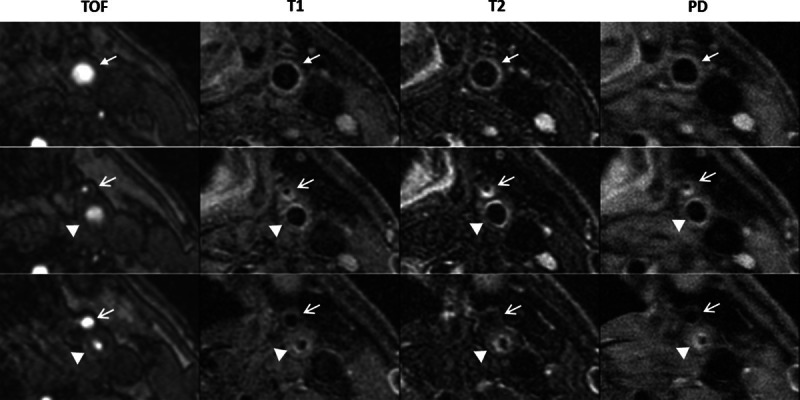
Multisequence MRI of the internal carotid artery at 3 different levels: first column; time-of-flight (TOF), second column; T1-weighted turbo-spin echo (T1), third column; T2-weighted turbo-spin echo (T2); and fourth column; proton density-weighted (PD). Top, Most caudal transaxial projection demonstrating *A. communis* (arrow). Middle, Intermediate transaxial projection demonstrating *C. interna* (arrowheads) and *C. externa* (open arrows). Bottom, Most cranial transaxial projection demonstrating *C. interna* (arrowheads) and *C. externa* (open arrows). Note the reduced blood flow on TOF in *C. externa* (middle) and *C. interna* (bottom). Plaque buildup can be seen in *C. externa* in T1, T2, and PD (middle) and in *C. interna* in T1, T2, and PD (bottom).
